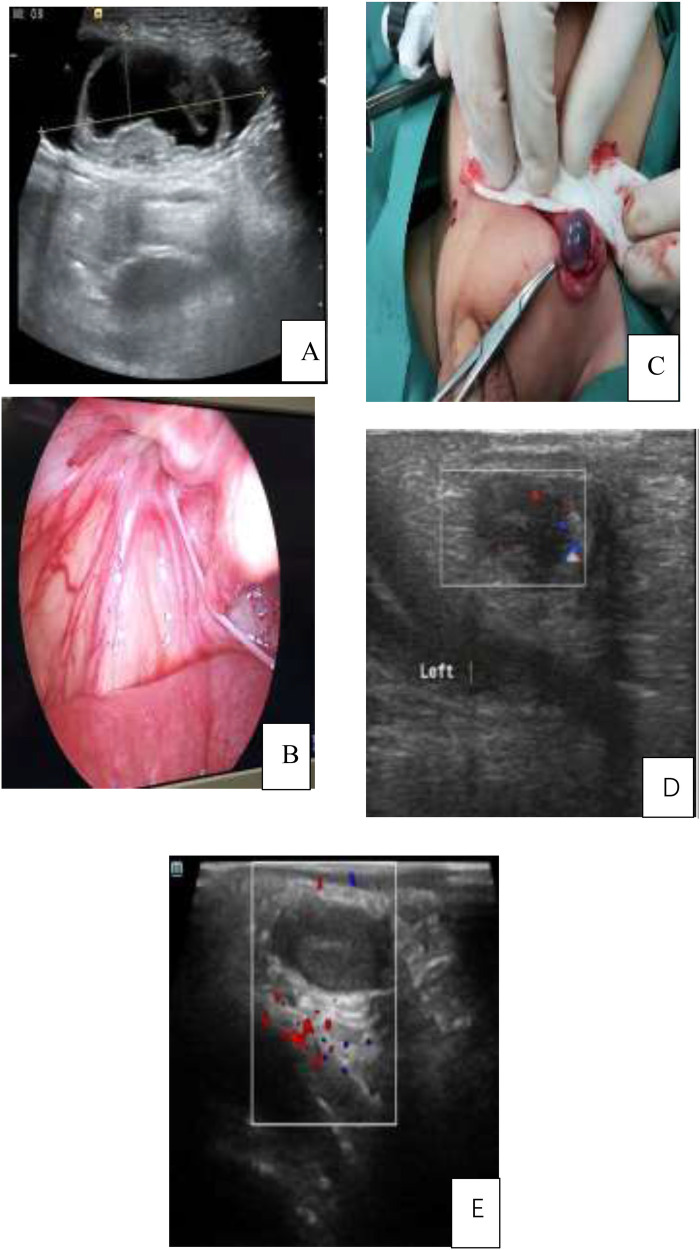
(A) The incarcerated contents in the left inguinal region were identified as bowel, with adjacent testicular tissue. (B) Successful reduction of the incarcerated bowel assisted by laparoscopy through an inguinal incision. (C) Intraoperative image showing partial congested testis without necrosis. (D) Postoperative day 3 Doppler ultrasound demonstrating testicular blood flow. (E) One-year follow-up ultrasound revealing partial testicular atrophy. Panel A: Ultrasound image showing a round structure with a dark center. Panel B: Endoscopic view of red tissue and vessels. Panel C: Surgical image of a gloved hand holding tissue with forceps. Panel D: Ultrasound with a rectangular overlay and colored markers. Panel E: Ultrasound with colored markers in a rectangular area.
